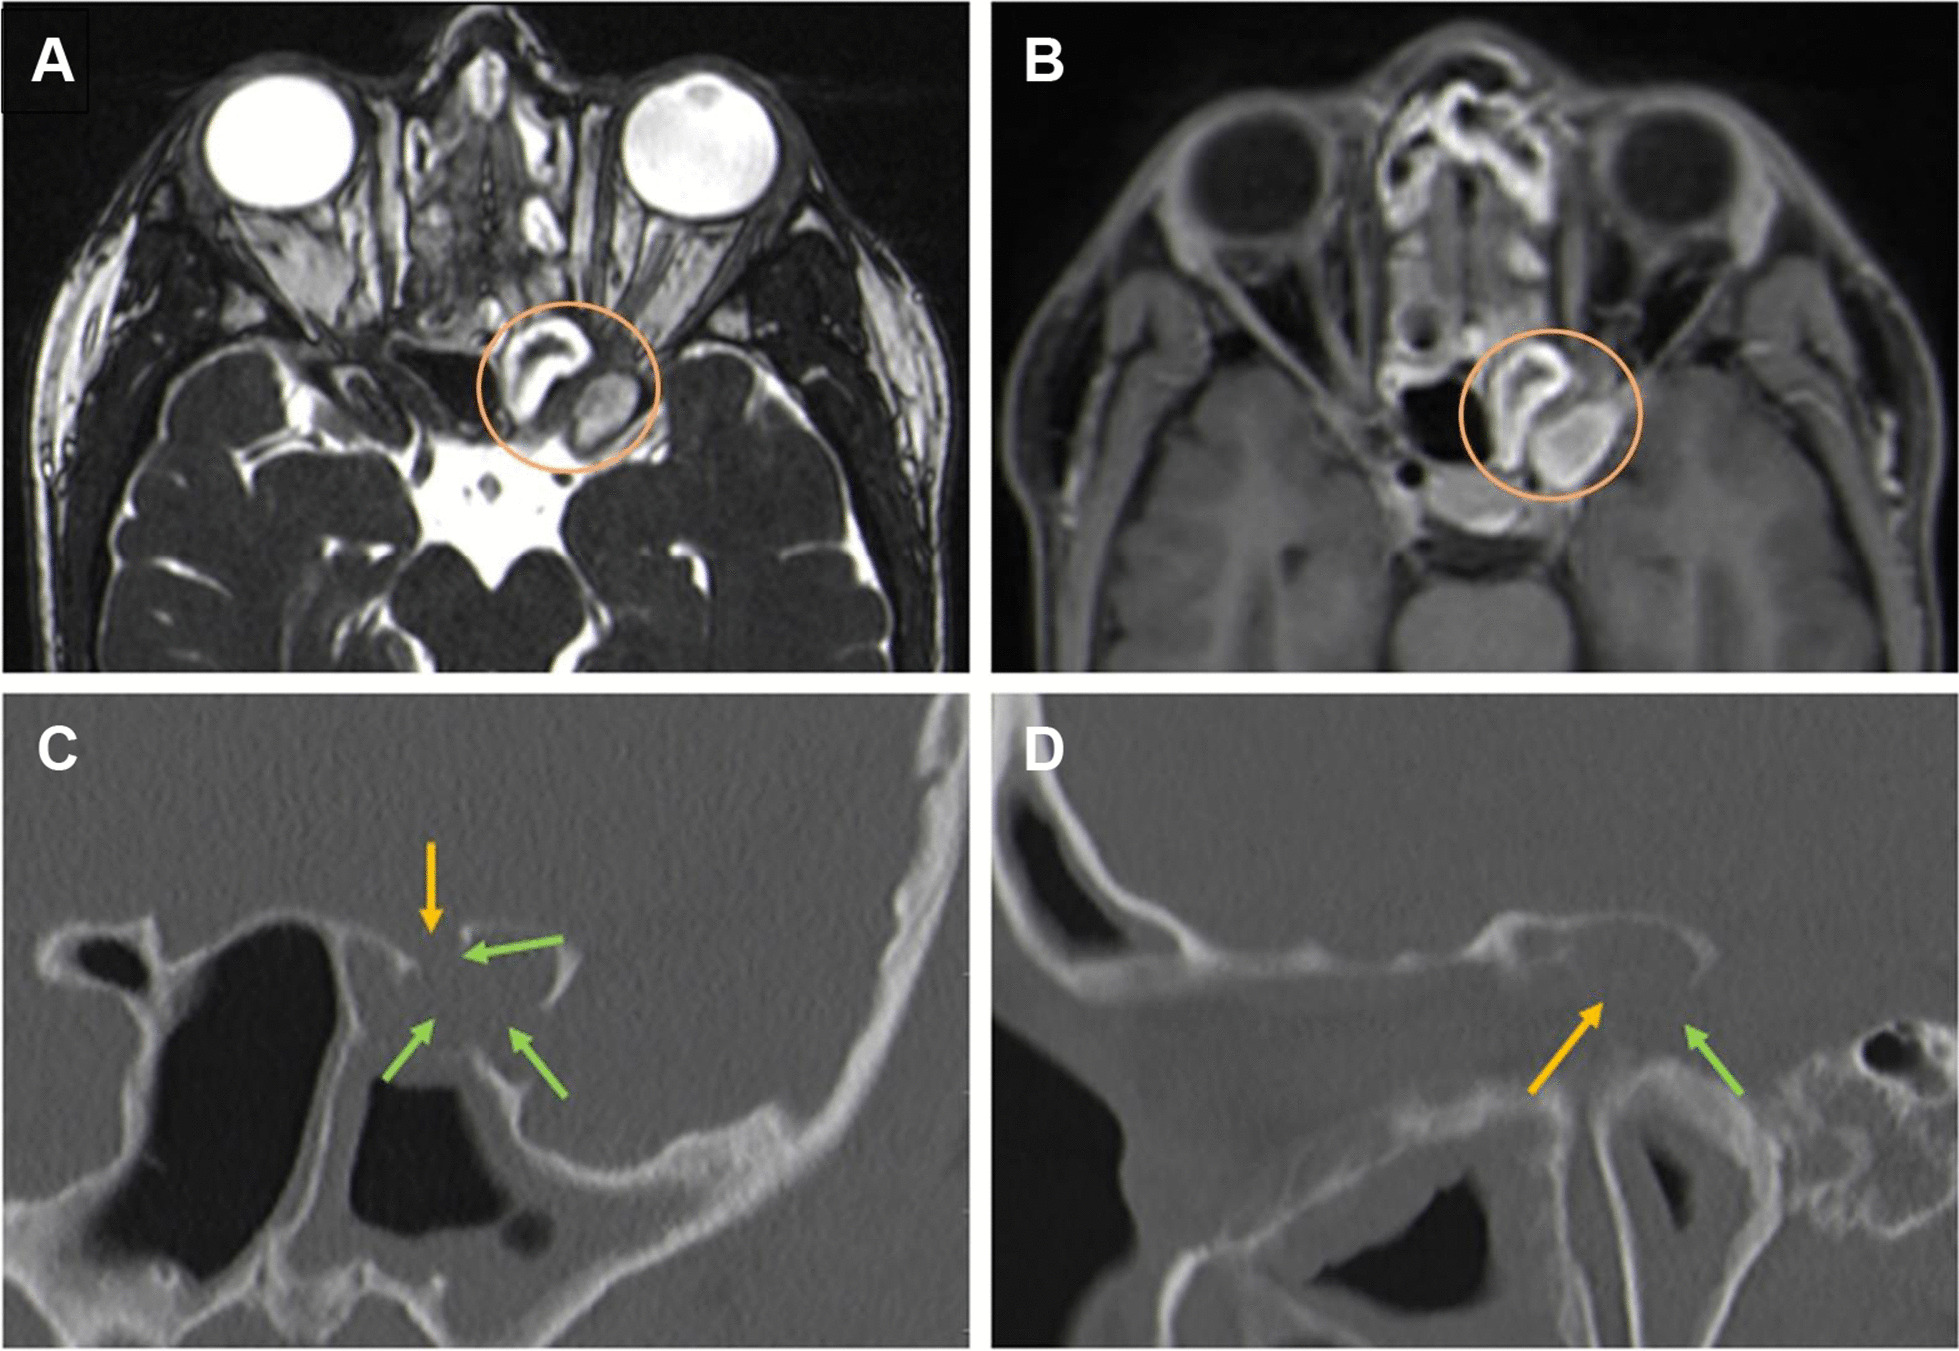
High-resolution cranial MRI and CT on admission. The high-resolution T2-weighted CISS images (A: axial plane) and contrast-enhanced T1-weighted SPACE FS (B: axial plane) show the course of the left optic nerve, with indentation and direct contact with the mucocele. No signs of optic nerve edema due to compression or ischemia are found (orange circle), supporting the additional presence of a local toxic impact. High-resolution CT (C: coronal plane; D: parasagittal plane) shows the shadowing of this Onodi cell, caused by a mucocele, as identified by the MRI sequences. Thinned bone lamellae or partial bone resorption (green arrows) and expanded bony margins are in direct contact with the course of the optic nerve (orange arrows)
